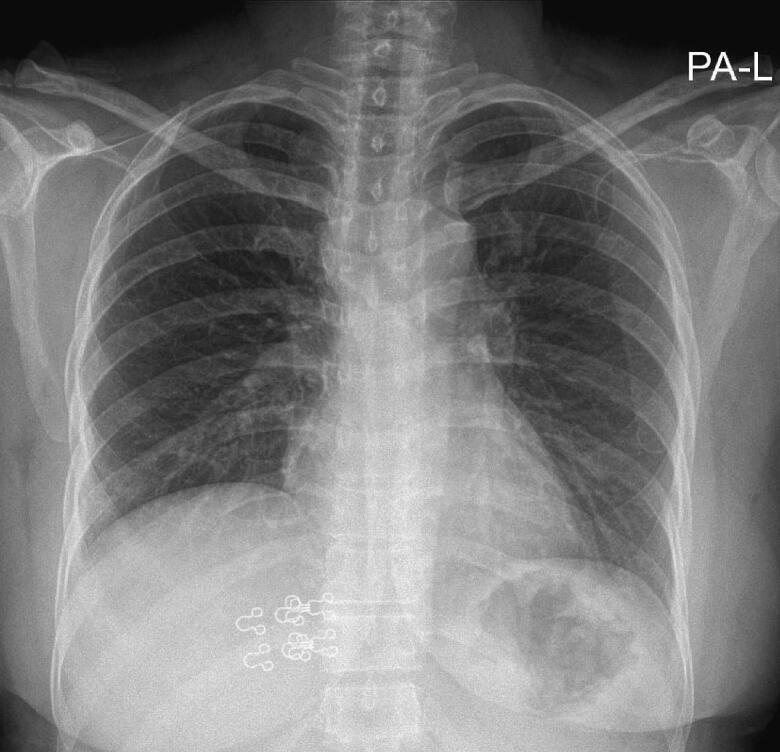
Initial normal chest X-ray.
